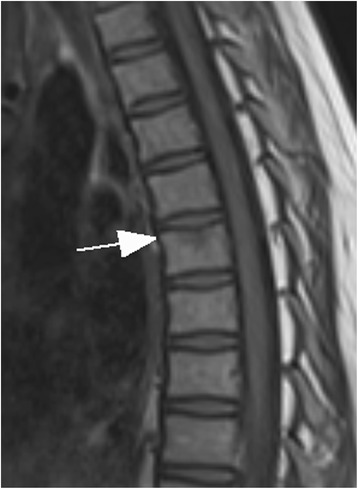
Shows the impression of the upper endplate of T6 vertebral in close up sagittal T1-weighted TSE MR imaging (*arrow*)
